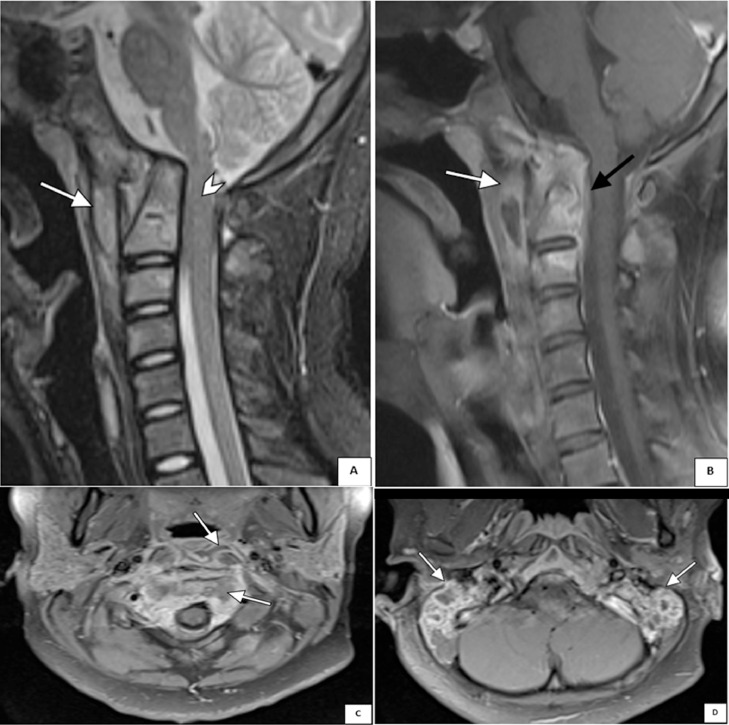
(A) Sagittal T2-weighted magnetic resonance image (MRI) of the cervical spine shows an abscess in the retropharyngeal space (white arrow) and stenosis of the spinal cord at the craniocervical junction level (arrowhead). (B) Sagittal contrast T1-weighted MRI image demonstrates a paravertebral abscess with posterior epidural involvement (white arrow), consistent with spondylitis (black arrow). (C) Axial contrast T1-weighted MRI image reveals an abscess in the retropharyngeal space extending to the spinal canal. (D) Axial contrast T2-weighted MRI image of the mastoid shows heterogeneous intensity of bilateral abscess.
